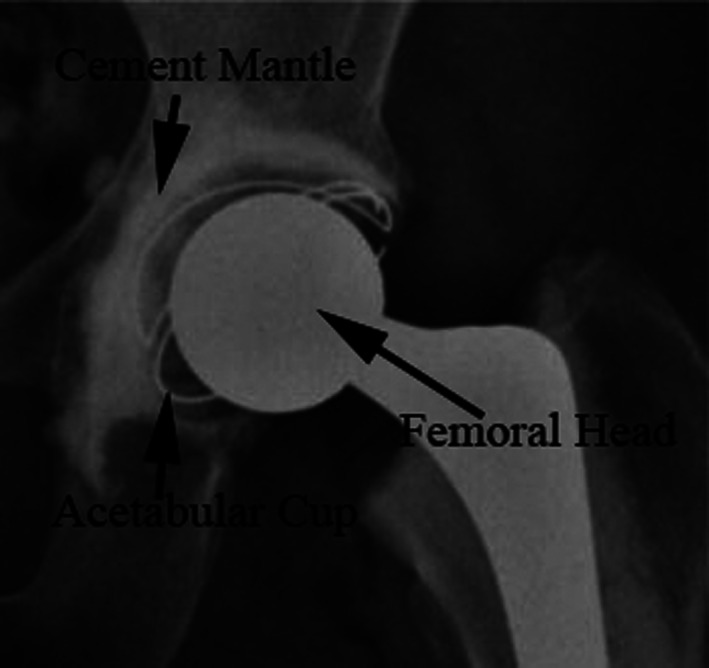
A radiograph of a cemented THR with annotations.^1^
